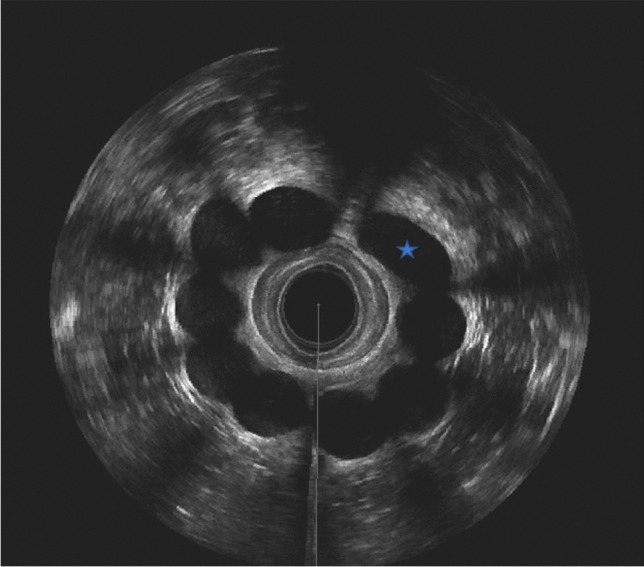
In the performed endoanal ultrasound, 9 prostheses are visible in correct localization in the intersphincteric space. However, one prosthesis is tilted (*) and thus classified as dislocated
